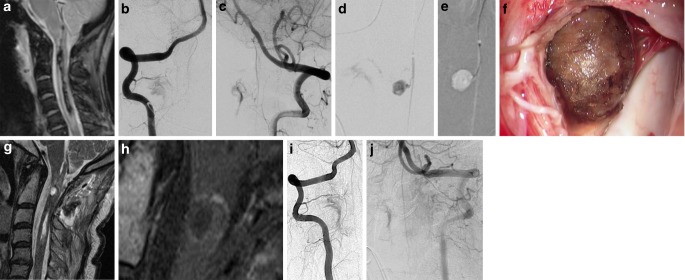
Diagnostic imaging and treatment in a patient with cervical hematomyelia due to an aneurysm of a sulco-commissural artery, associated with a cervical intradural arteriovenous fistula. T2*weighted image showing the hematoma in the cervical spinal cord and the surrounding edema (a). DSA at this time revealed an intradural arteriovenous fistula with dominant radiculomeningeal supply via the right VA (b). Injection of the left vertebral artery shows the pial supply of the intradural arteriovenous fistula via the anterior spinal artery (c). Selective injection of the anterior spinal artery 5 months later shows the connection between the anterior spinal artery and the aneurysm via a sulco-commissural artery (d). Meanwhile the aneurysm had considerably increased. While the guidewire could be introduced into the sulco-commissural artery, the vessel’s small caliber did not allow passage of the microcatheter (e). Via a small microsurgical approach to the cervical spinal cord from the right side and after dissection of the spinal cord tissue, the wall of the aneurysm became visible (f). Thermocoagulation of the wall of the aneurysm resulted in shrinkage of the lesion, providing additional space for further dissection of the whole aneurysm. After mobilization of the aneurysm, the afferent sulco-commissural artery was identified and the borders of the adjacent spinal cord were visible. The feeding artery was divided with microscissors. Postoperative follow-up MRI on the first day after the microsurgical aneurysm resection shows the hemosiderin remnants (T2-weighted image g) while the previous contrast enhancing aneurysm wall is no longer visible on contrast enhanced T1WI (h). DSA of the right (i) and left (j) vertebral artery 3 weeks after the microsurgical resection of the sulco-commissural artery aneurysm. The previous aneurysm is no longer visible, the continuity of the anterior spinal artery is preserved and a small remnant of the intradural AV-fistula remains perfused
